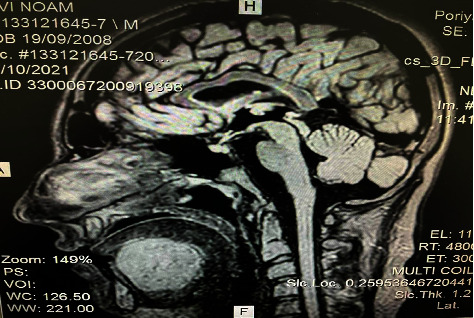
Sagittal MRI scan showing a homogeneous cystic lesion measuring 5.4 *∗* 3.1 *∗* 4.6 cm with a delicate capsule and uniform high-protein content liquid. Peripheral enhancement is observed after gadolinium injection in the sublingual space.
